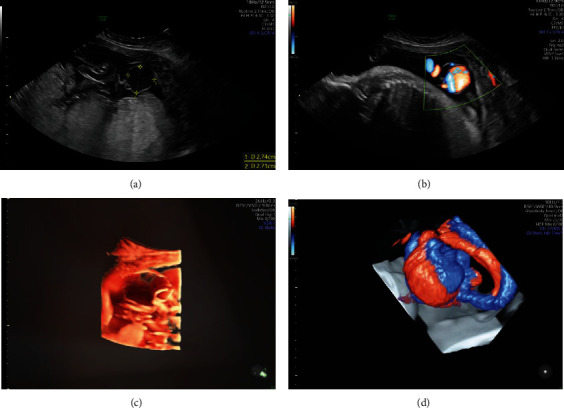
(a) Top left—2.7 cm umbilical vein aneurysm free-floating cord. (b) Top right—color doppler demonstrating bidirectional flow within umbilical vein aneurysm. (c) Bottom left—high definition live 3D rendering of umbilical vein aneurysm. (d) Bottom right—color 3D rendering of umbilical vein aneurysm.
